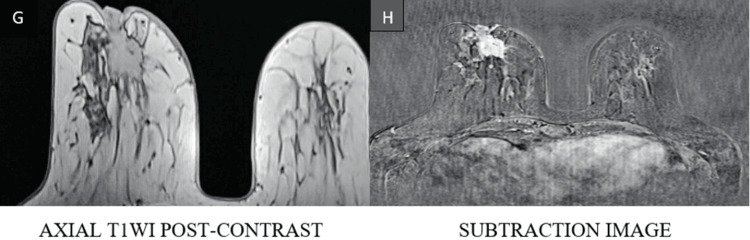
Right breast lesion demonstrates avid heterogeneous enhancement on post-contrast imaging (G, H) T1WI: T1-weighted image
